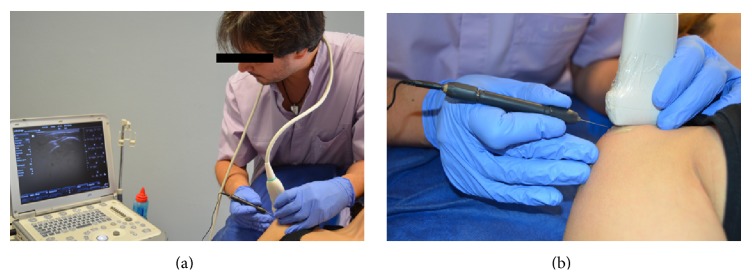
Application of US-guided percutaneous electrolysis on a real patient with the clinician following the application on the ultrasound screen (a) and a detail of the application (b).
